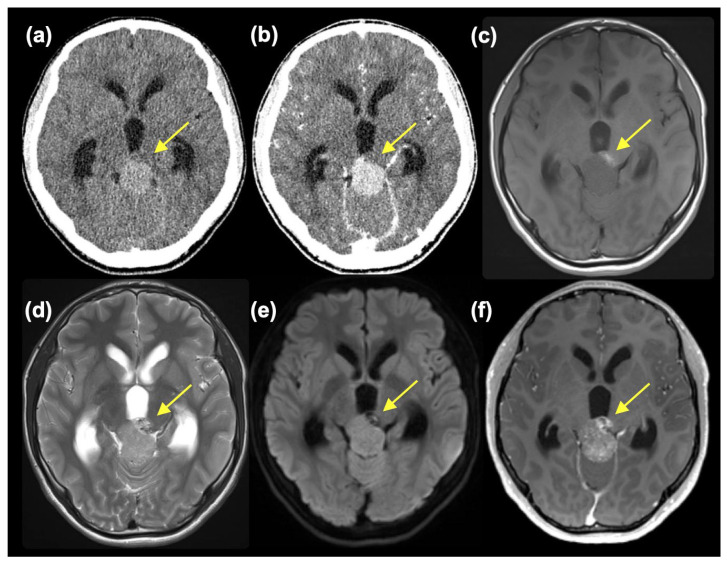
Pre-contrast (a) and post-contrast CT (b) and T1WI (c), T2WI (d), DWI (e), and post-contrast MRI (f) showing two components (small component indicated with yellow arrow).
